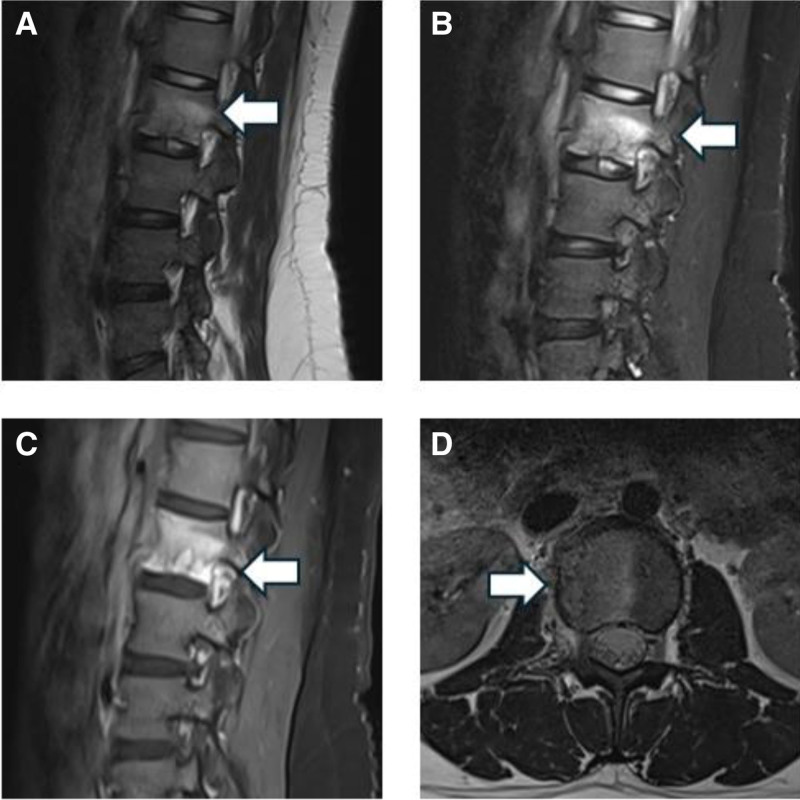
MRI images obtained before admission. Sagittal Gd-enhanced T1-weighted (A), T2-weighted (B), T2-weighted fat-saturation (C), and axial Gd-enhanced T2-weighted images (D) showed patchy abnormal signal shadows on the L2 vertebral body. MRI = magnetic resonance imaging.
